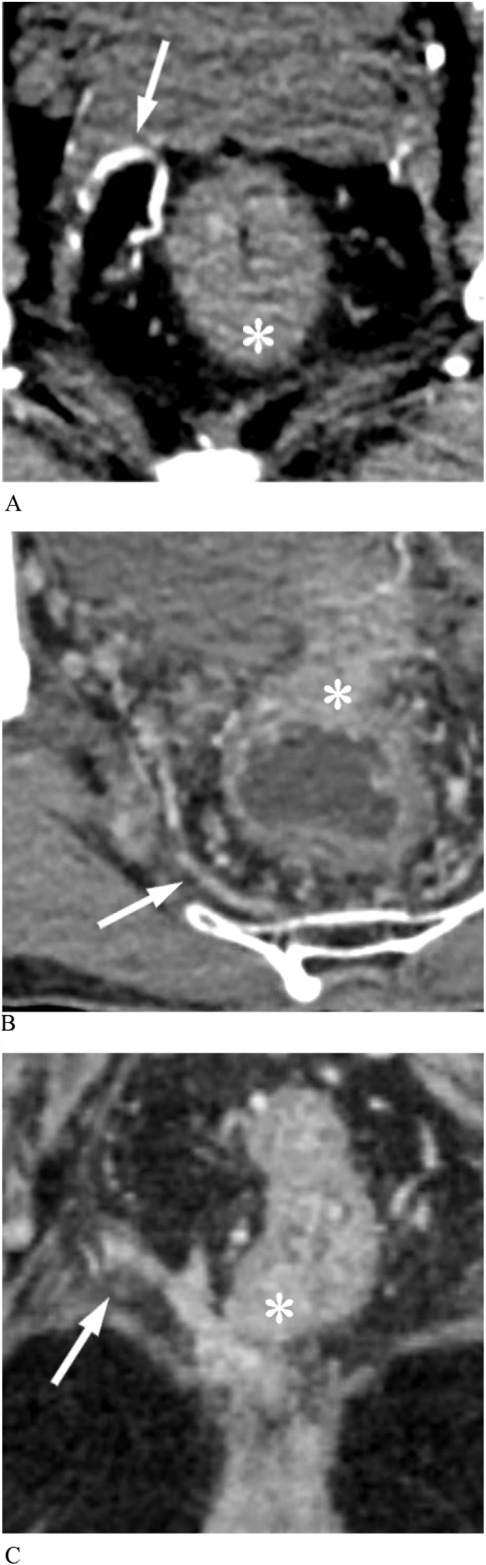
Middle rectal arteries (MRAs) display on enhanced CT images. (A) Arterial phase image shows that an anterolateral MRA (arrow) penetrates the mesorectal fascia into the rectal tumor (asterisk). (B) Venous phase image shows a posterolateral MRA (arrow) communicating pelvic plexus with the rectal tumor (asterisk). (C) The rectal cancer (asterisk) has a protrusion of stripes connected to the pelvic vessel branch, representing MRA (arrow).
